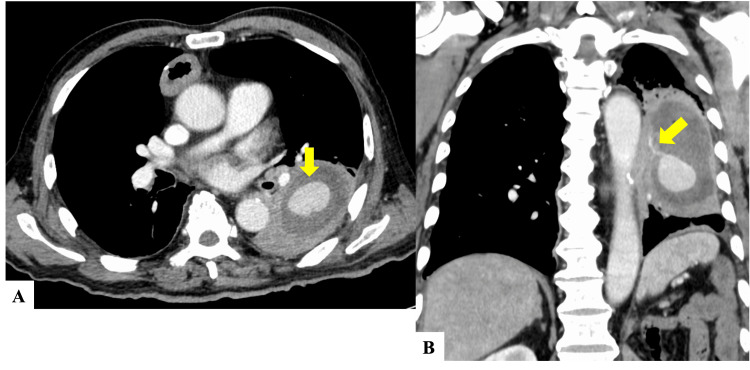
(A) Axial contrast-enhanced chest CT shows an enhanced nodule (PAP) in the lung abscess (arrow). (B) In coronal reconstruction, a vessel feeding into the PAP is observed (arrow). PAP: Pulmonary artery pseudoaneurysm
